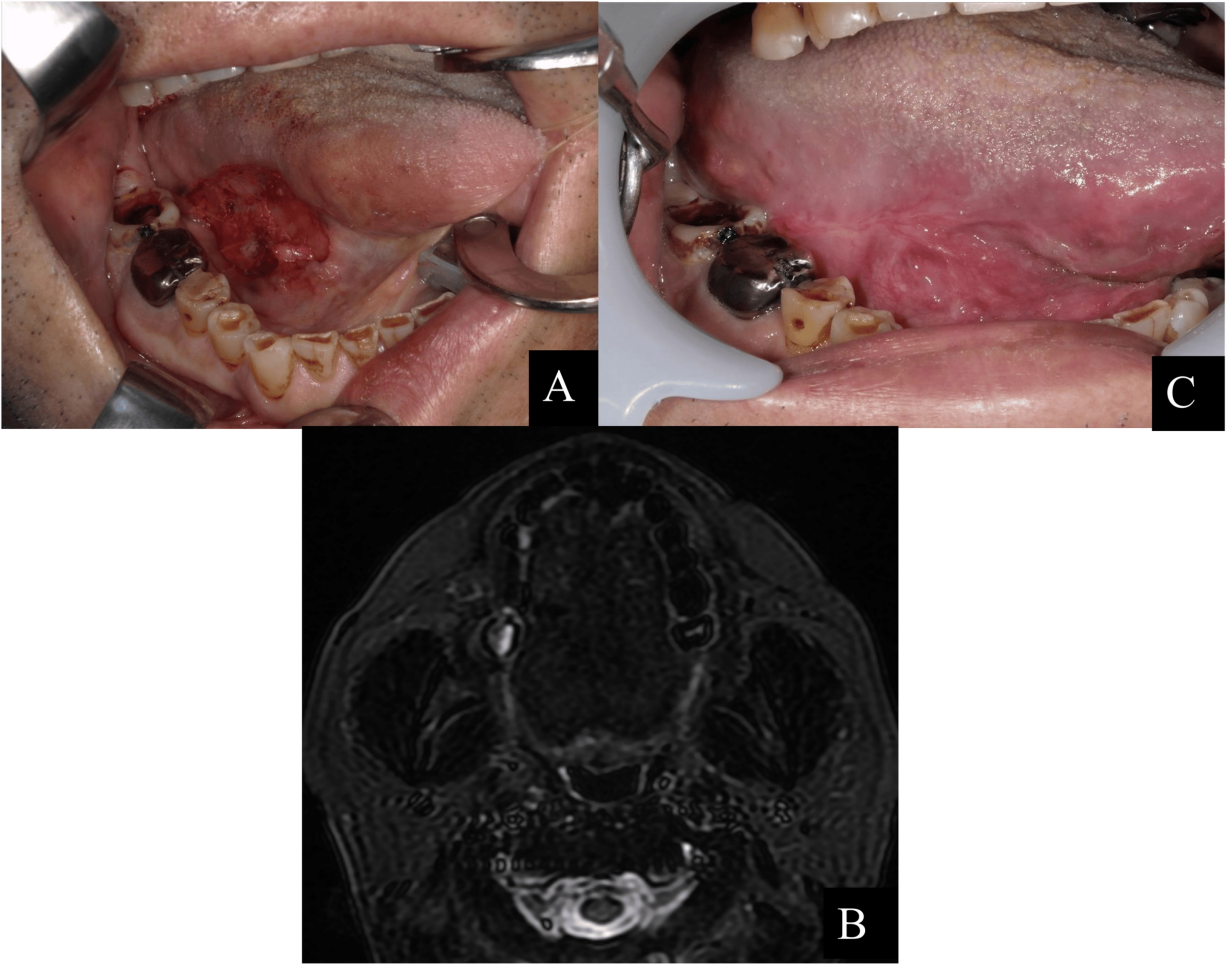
Treatment process of marsupialization for intraoral ranula. (A) An intraoperative intraoral photograph was taken 14 months after the initial visit (12 months after tongue cancer surgery). The ranula marsupialization was performed under general anesthesia. (B) A contrast-enhanced MRI, T2-weighted image was taken 17 months after the initial visit (three months after ranula marsupialization). No recurrence of either ranula or tongue cancer was noted. (C) An intraoral photograph was taken 26 months after the initial visit. No recurrence of either ranula or tongue cancer has been noted to date.
